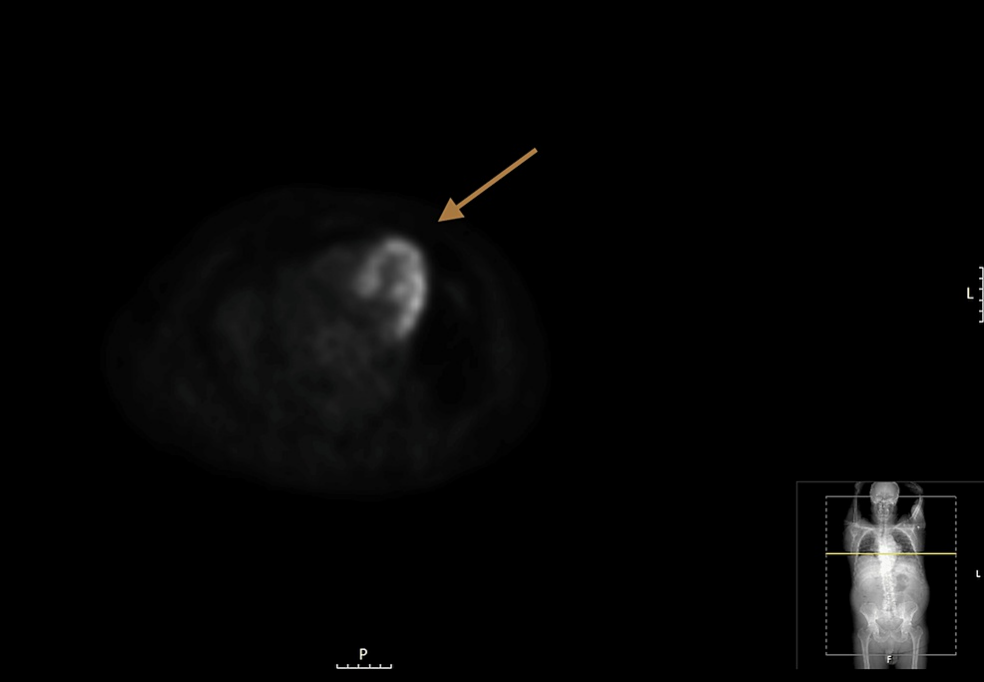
PET-CT scan, axial view, showing the anterior mediastinal mass with moderate-to-intense avidity for FDG. PET-CT, positron emission tomography computerized tomography; FDG, fluorodeoxyglucose
